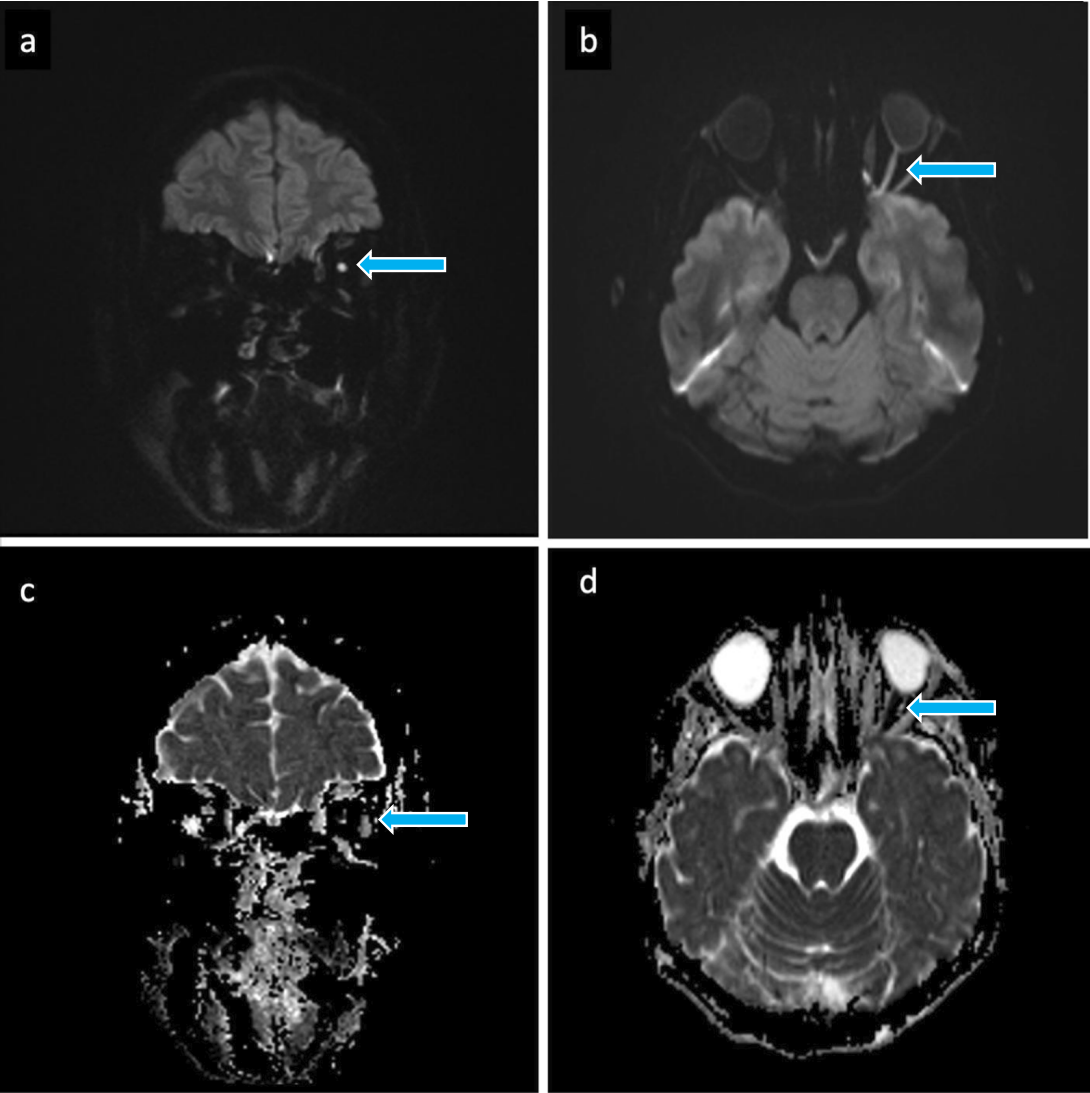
MRI of the brain 1.5 weeks after the HA filler injection. Coronal (a) and axial (b) diffusion weighted imaging (DWI) b = 1000 images and corresponding apparent diffusion coefficient (ADC) maps (c, d) highlighting more pronounced signal changes in the left optic nerve
